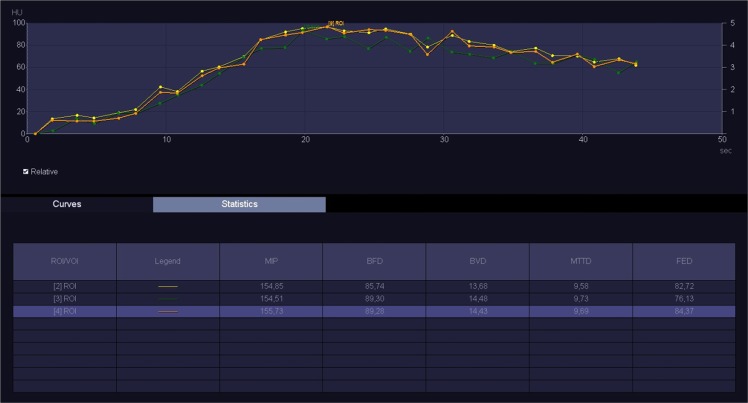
Perfusion CT: curves for blood flow, blood volume, mean transit time and time to peak.
